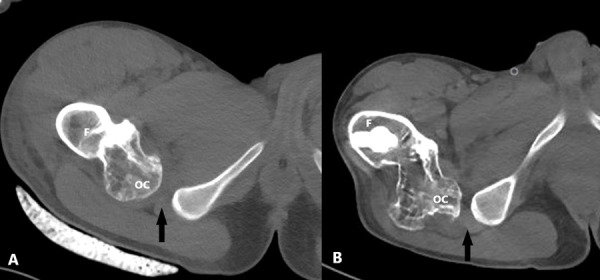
The axial hip CT image taken after trauma in the emergency department (before the operation) (A) and control axial CT image after six months from the operation (B). After the treatment, the *ischiofemoral distance* narrowed and the compression of the *quadratus femoris muscle* increased on CT (*ischiofemoral distance* indicated by black arrows). *F: Femur, OC: Osteochondroma*.
